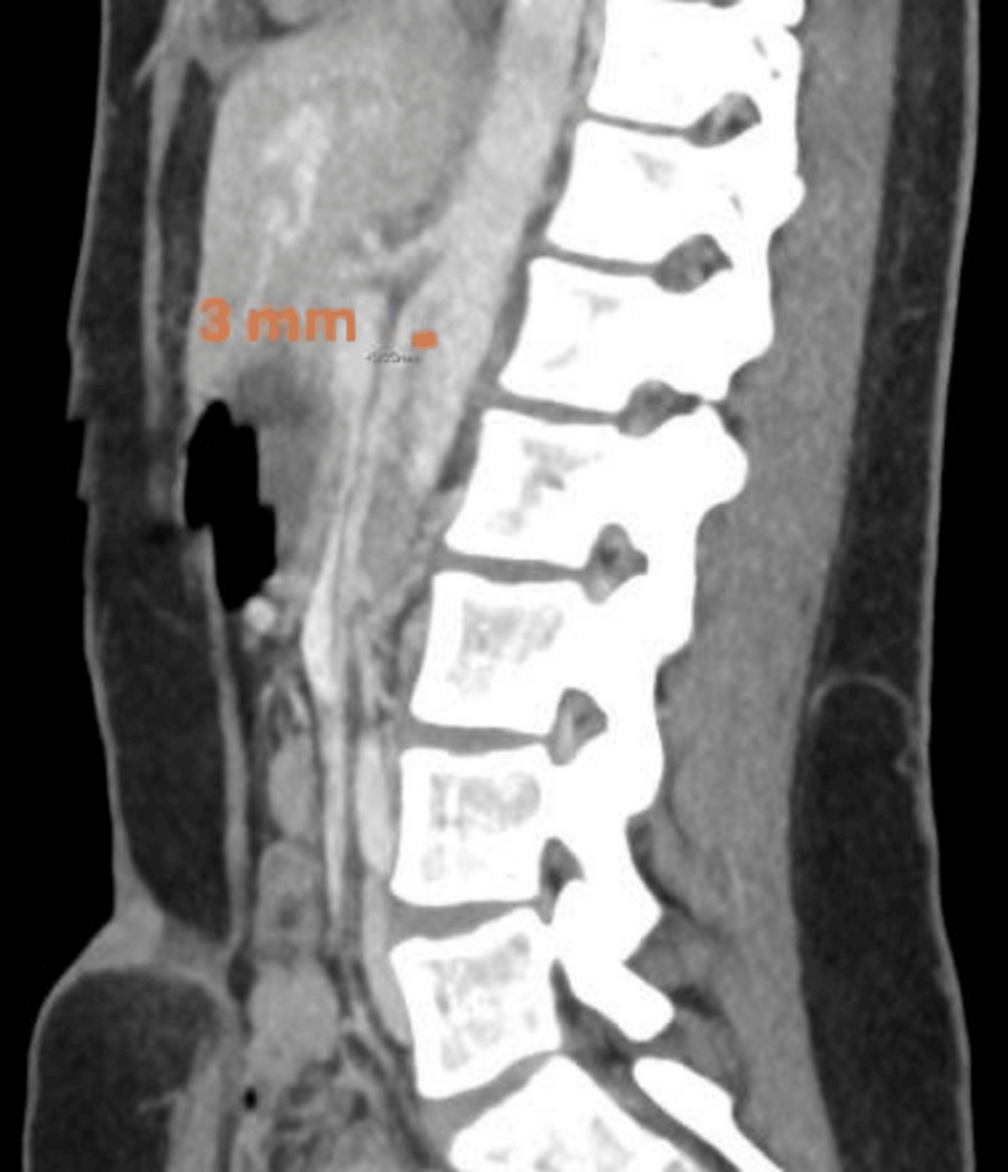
Sagittal CT abdomen slice used to evaluate for aortomesenteric distance measures 3 mm (orange bar), in keeping with superior mesenteric artery syndrome.
